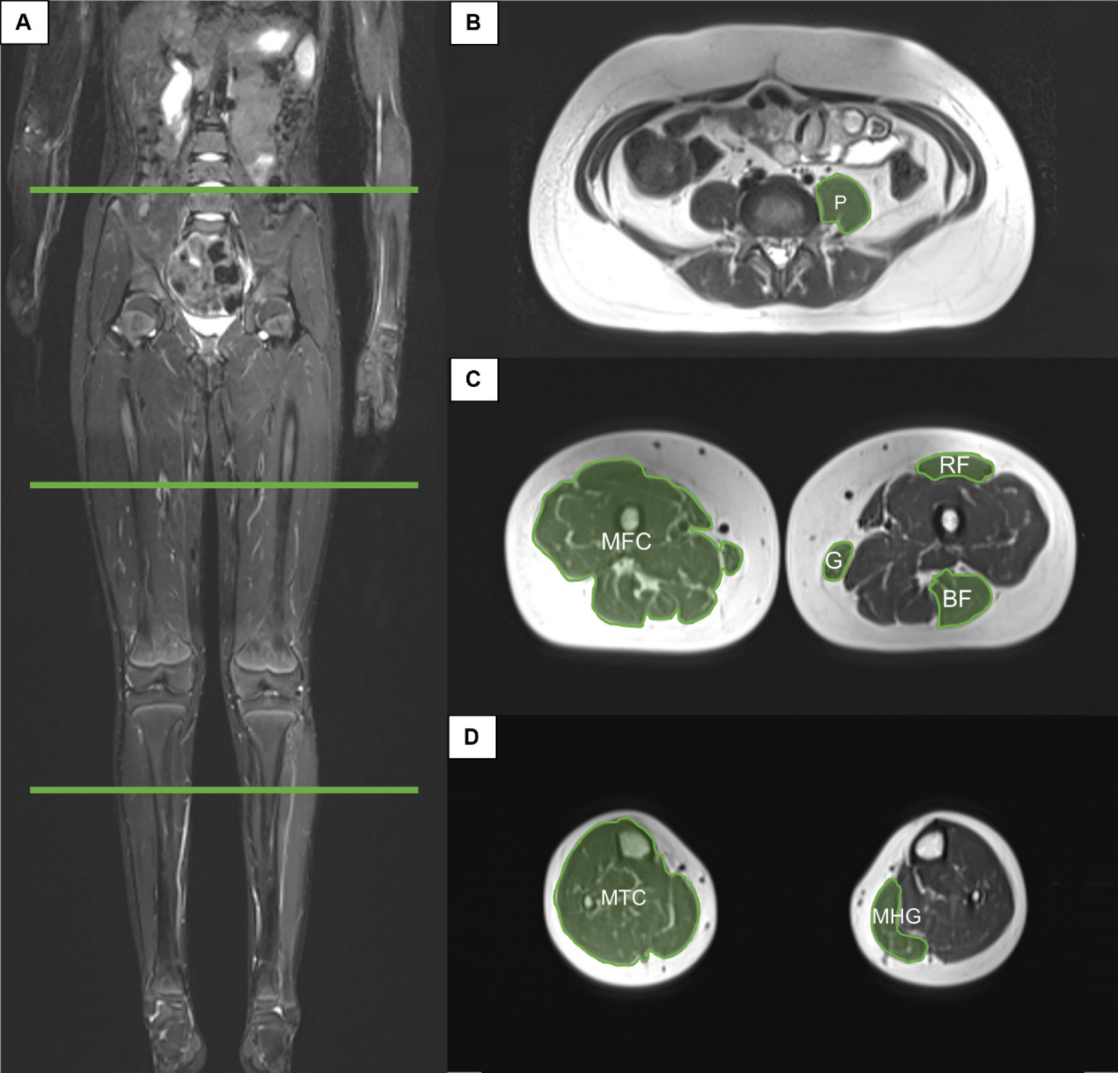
MRI measurements of muscle cross‐sectional area showing (A) coronal view with level for segmentation identified (L3/4 intervertebral disc, 50% length of the femur and 33% length of the tibia). (B) Axial view of psoas muscle; P, Psoas muscle. (C) Axial view of thigh muscles; B, Biceps femoris; G, Gracilis; MFC, Mid‐femoral muscle circumference; RF, Rectus femoris. (D) Axial view of calf muscles; MHG, Medial head of gastrocnemius; MTC, Mid‐tibial muscle circumference.
